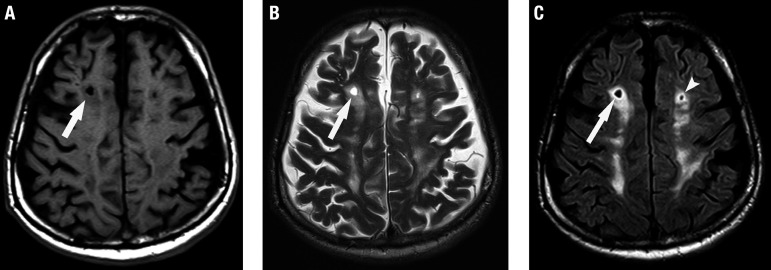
Lacune of presumed vascular origin. A 66-year-old patient with vascular dementia. Axial T1-weighted (A), T2-weighted (B) and FLAIR (C) images show the lacune in the deep white matter of the right centrum semiovale (arrows), with signal intensity similar to CSF in all sequences, with a peripheral rim of gliosis, best seen on FLAIR image (C). Note the concomitance of white matter hyperintensities of presumed vascular origin on FLAIR (C) and T2-weighted (B) images, with corresponding hypointensity on T1-weighted image (A). There is also another lacune in almost the same location, but in the left centrum semiovale (arrowhead in C).
